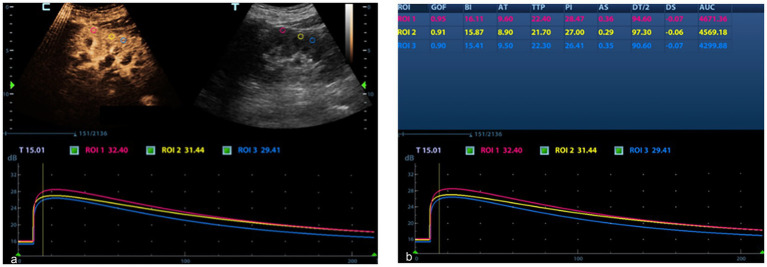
TIC of a reflux obstruction renal contrast enhancement. (a) Cortical sampling site and corresponding TIC. The screen displays the contrast-enhanced image (C) alongside the conventional ultrasound image (T). (b) Plot of ROIs analyzed by dedicated software. The patient was a 53-year-old male diagnosed with heart failure, pulmonary edema, and shock. CEUS parameters were characterized by markedly decreased AS and prolonged DT/2. These findings may indicate impaired venous outflow and prolonged microvascular washout, potentially associated with venous congestion–related renal perfusion abnormalities.
